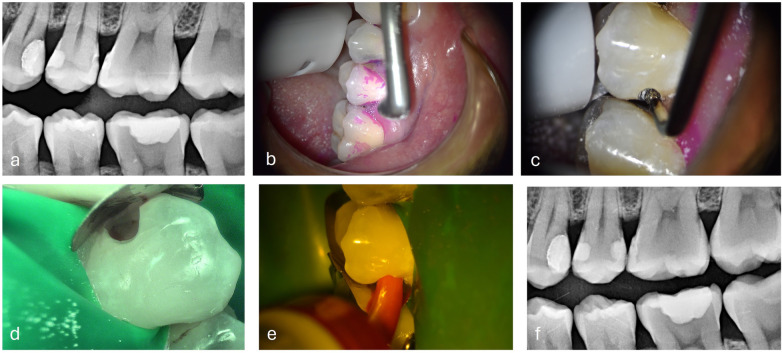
Slot cavity on a distal carious lesion of an second upper premolar. (a) Bitewing x-rays (b) Cleaning step with airflow, EMS dye plaque (c) US diamond insert half-round, (NSK®, Japan) (d) Slot preparation with metal matrix setting, intraoral C50® camera view (Acteon, France) (e) HVGIC LC injection (Riva® SDI, Australia), (f) Bitewing x-rays control.
